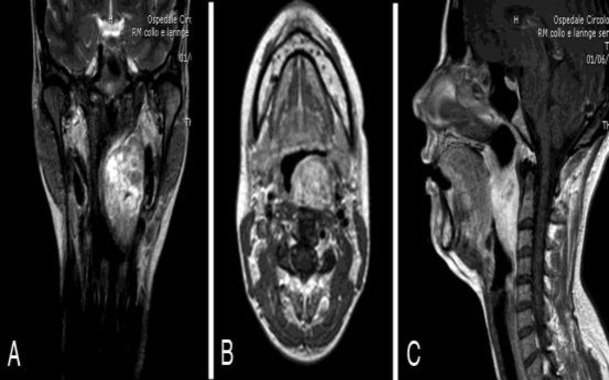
Preoperative MR shows a large mass arising in the left retropharyngeal space with bright signal intensity on T2-weighted images in coronal view (A) and a strong enhancement after gadolinium administration on T1-weighted images in axial view (B) and sagittal view (C).
